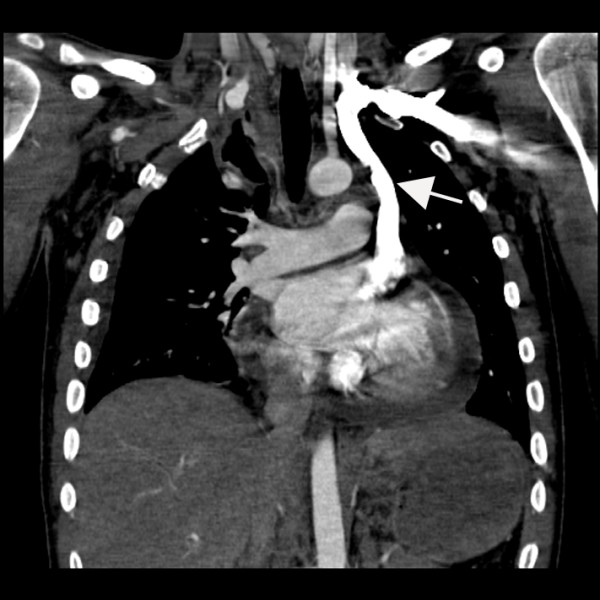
CT thorax showing left sided superior vena Cava (large arrow).
